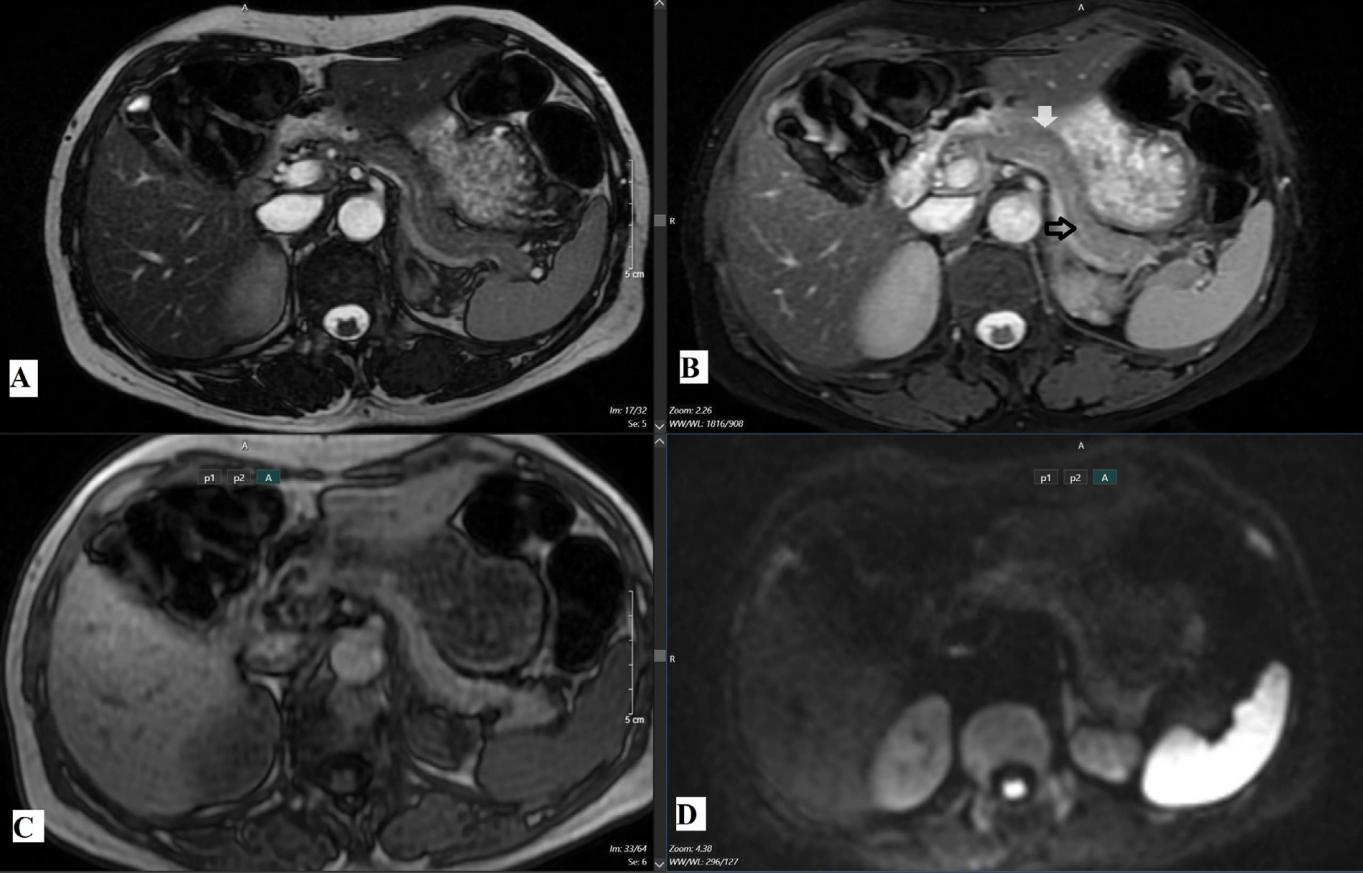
Magnetic resonance imaging (MRI) sequences of the pancreas, assessing the body (white arrow) and tail (black arrow): (A) T2-weighted image (T2W), (B) diffusion-weighted image at b1000 (DWI-b1000), (C) T2-weighted fat-saturated image (T2W fat sat), and (D) T1-weighted in-phase image (T1W in-phase).
